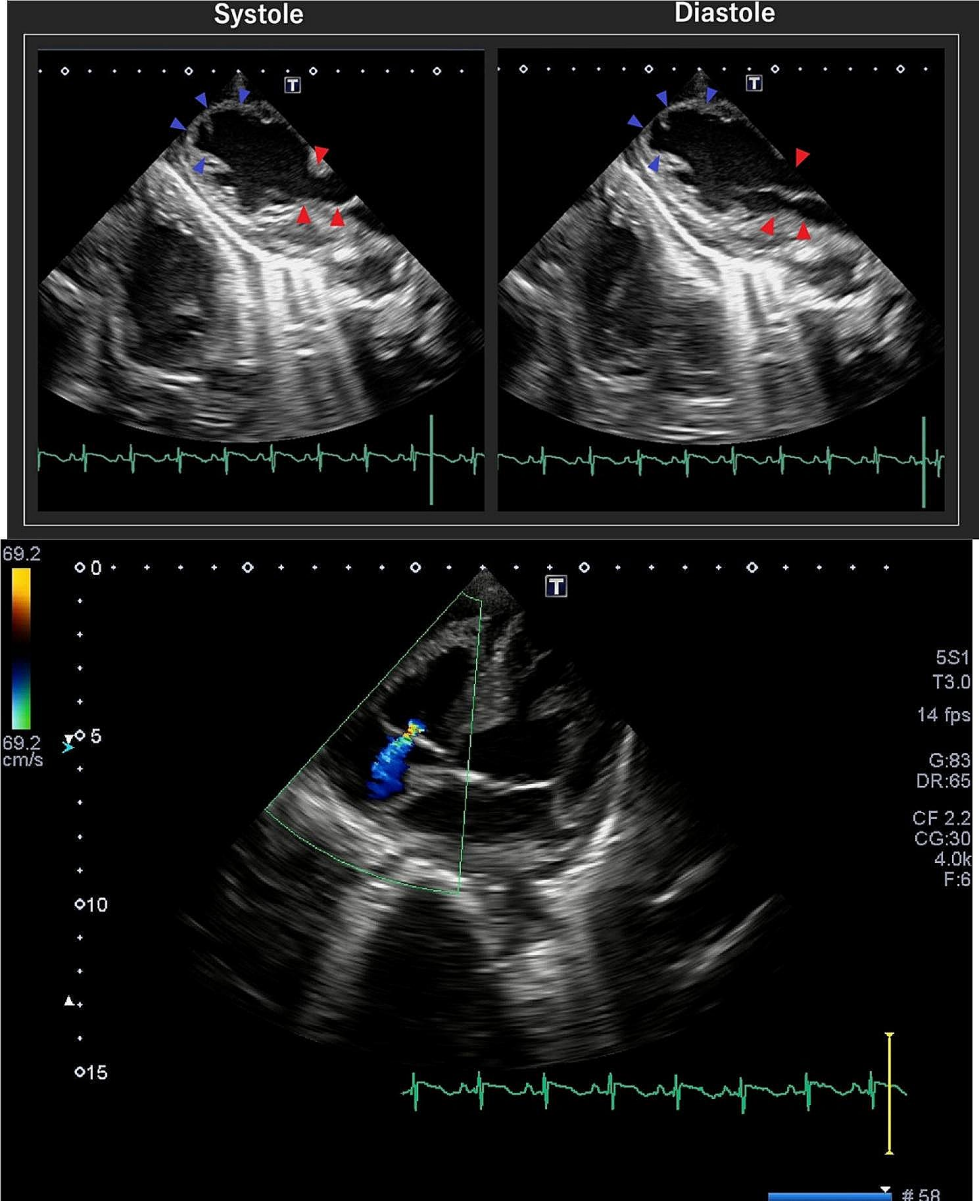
Echocardiogram of the case. The red arrow shows the hyperkinesis of the basal walls and the blue arrow shows the akinesis of the apex
